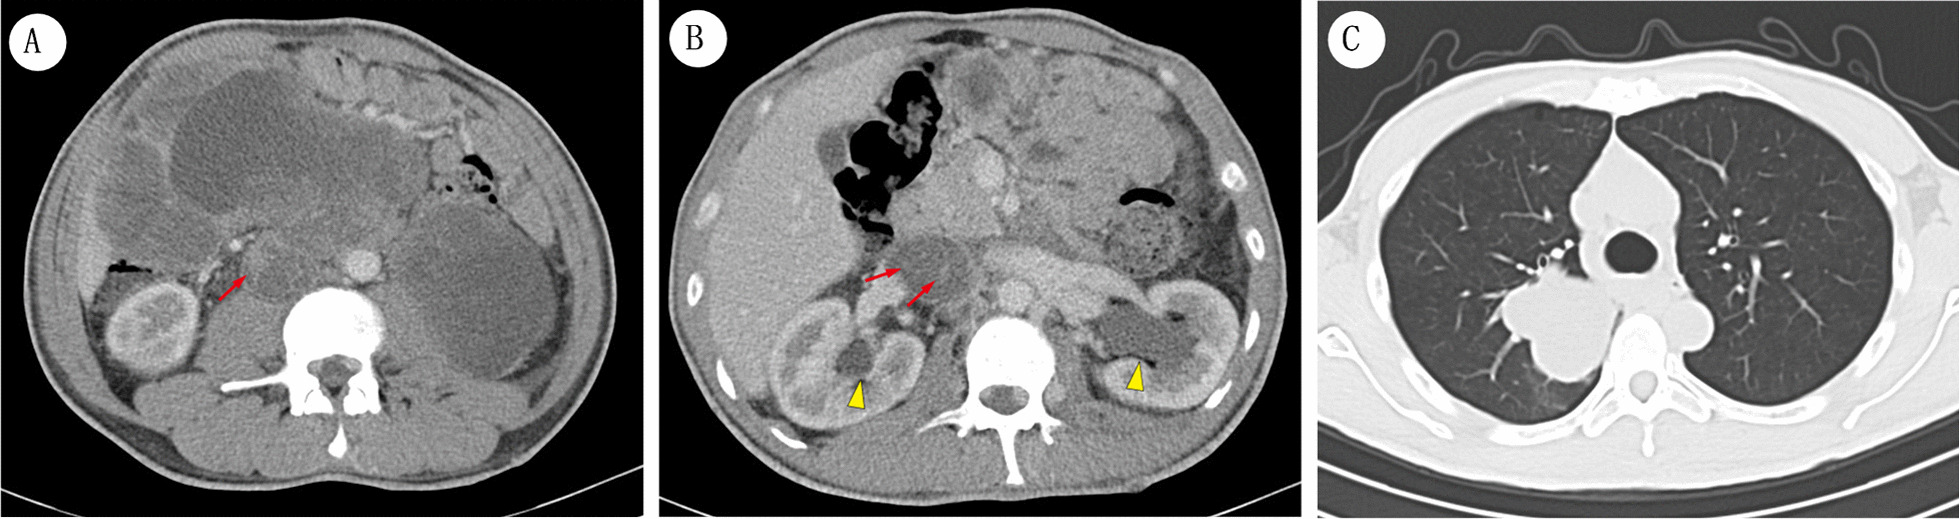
CT images of the 37-year-old male, five months later after radical mass excision. A Contrast-enhanced abdominal CT scan shows multiple irregular nodules and masses in abdominal cavity and retroperitoneal spaces. Tumor embolus was distinct in postcava (*red arrows* in A and B). B It is observed that reappeared mass grows into the perirenal spaces and blocks ureters on both sides, which give rise to pelvicalyceal dilatation and hydronephrosis (*yellow arrows*). C Chest CT scan demonstrates a huge well-defined mass appears adjacent to the right-side pulmonary hilum
